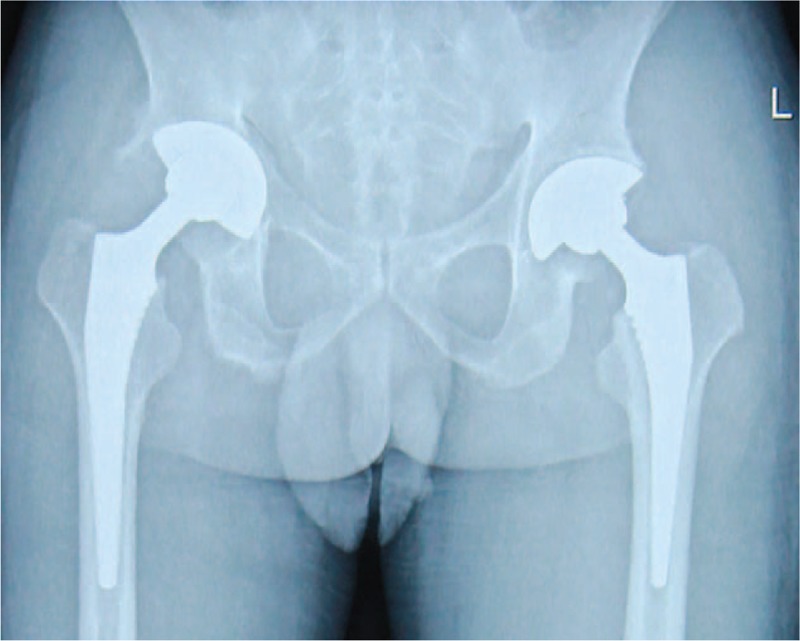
Postoperative anteroposterior radiographs of the patient 1 week after cementless THA. THA = total hip arthroplasty.
